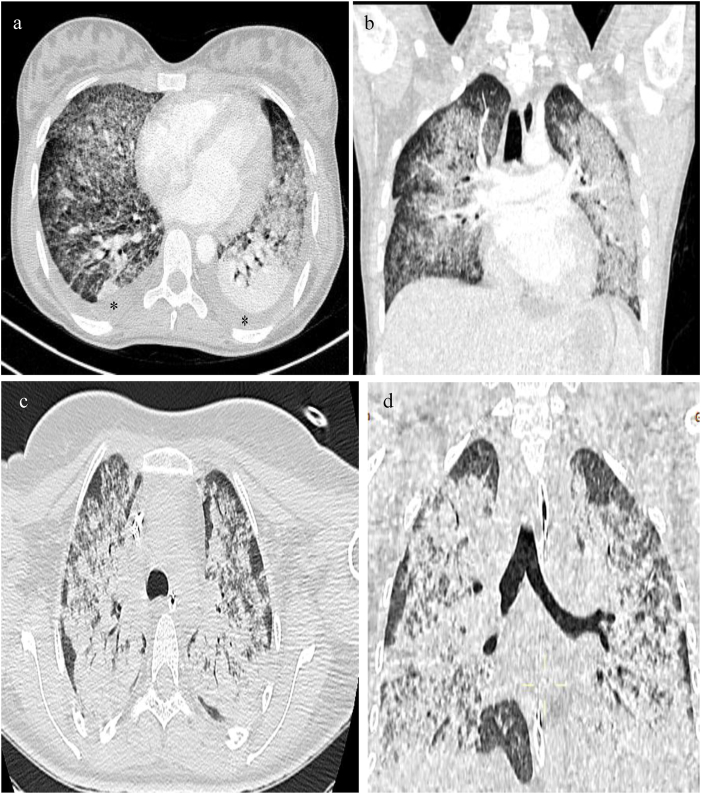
‘Indeterminate Covid-19 appearance’ Chest CT images in 2 patients showing ground glass opacities and consolidations with no specific distribution nor morphology in a case of.*(a,b) Acute eosinophilic pneumonia associating interlobular septal thickening, lower lobes air space consolidations and pleural effusions (*).(c,d) Extensive, multifocal, bilateral consolidation in a case of diffuse alveolar hemorrhage.*
